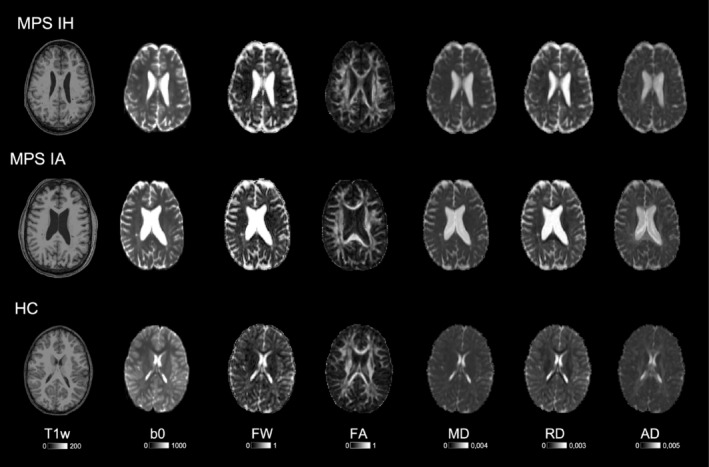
Example MRI data of MPS IA, MPS IH patients, and healthy control (HC). Showing anatomical T1‐weighted (T1w), diffusion‐weighted scans (b0), free‐water fraction (FWF), and free‐water corrected diffusion maps of fractional anisotropy (FA), mean (MD), radial (RD), and axial diffusivity (AD).
